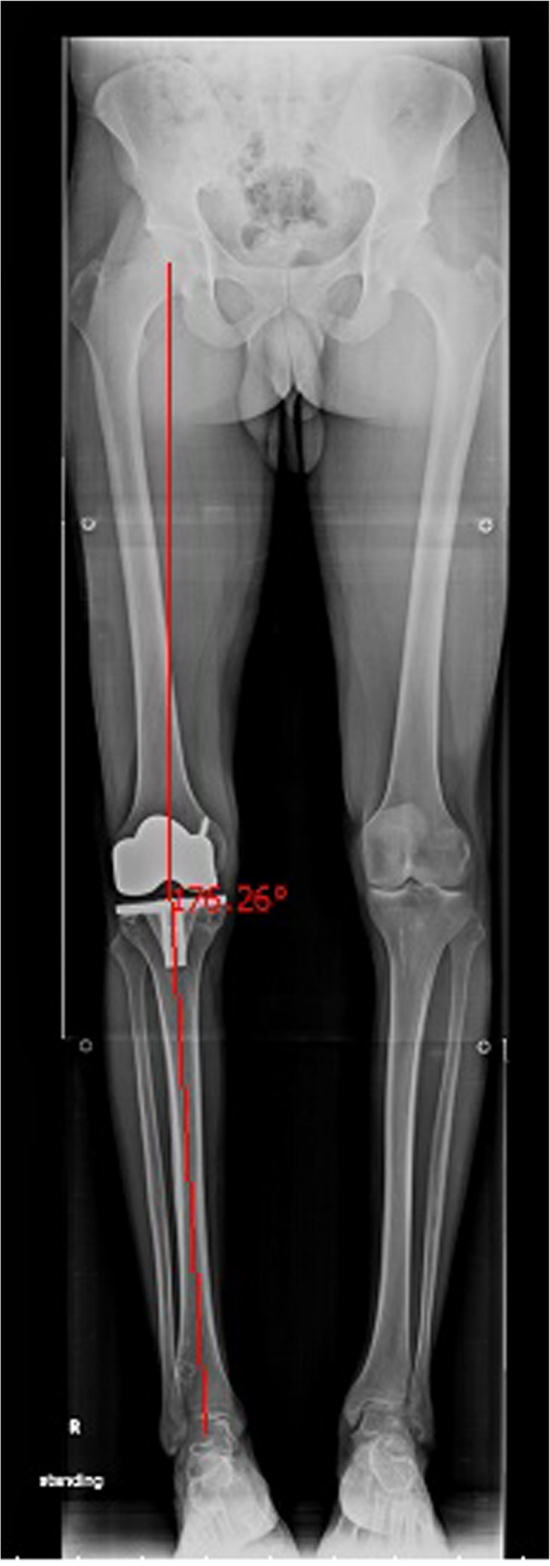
Full-length, standing-position, anterior-posterior radiograph of both lower limbs showing the hip-knee-ankle (HKA) axis
